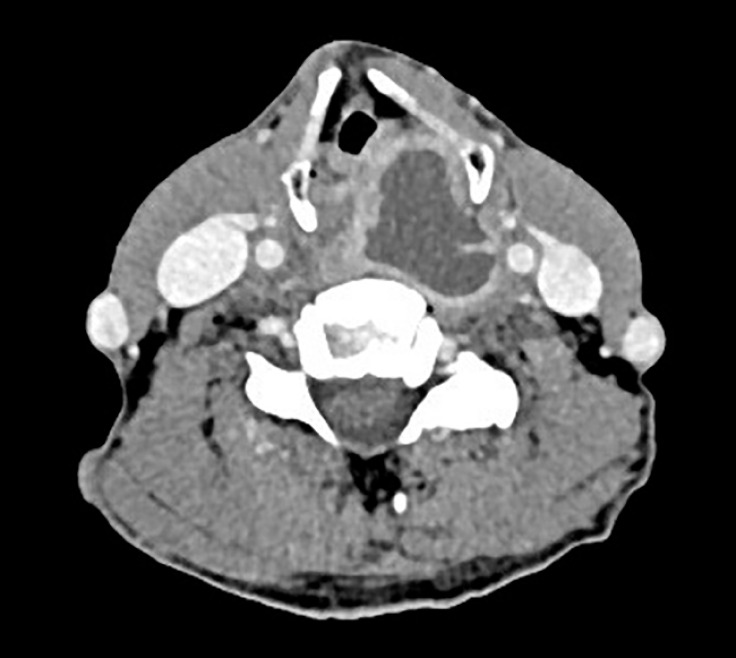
Computed tomography image, showing left retropharyngeal abscess with suspected separated diaphragm.
